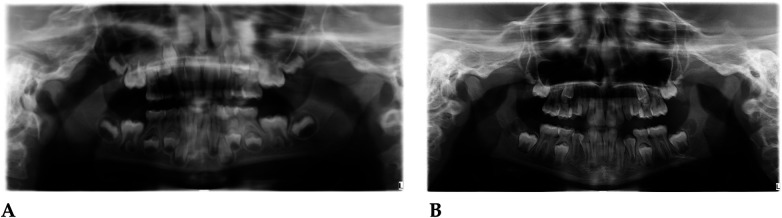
Pre- (A) and post-treatment (B) panoramic radiographs of case 3.
